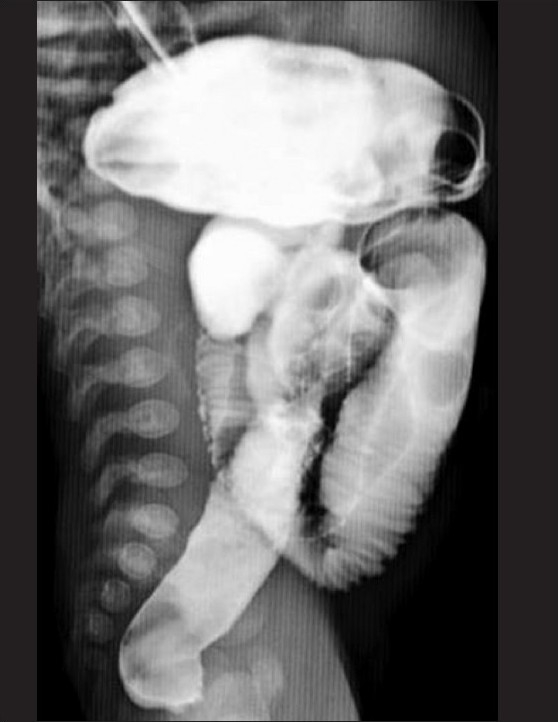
Lateral radiograph from a barium meal follow-through study shows the short length of small bowel with rapid transit (contrast is noted in the entire gastrointestinal tract, from the stomach to the rectum)
